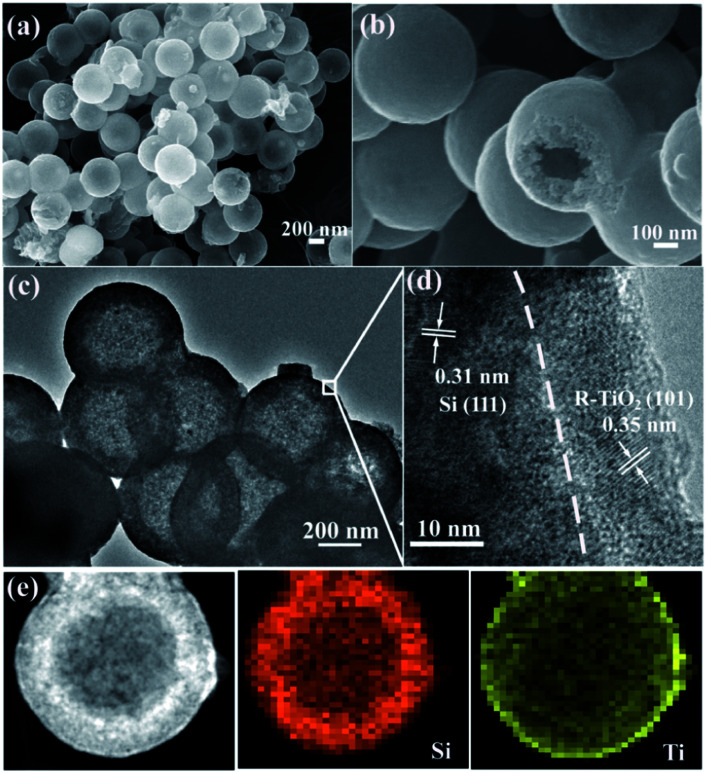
(a and b) SEM images and (c and d) HRTEM images of MHSi@TiO_2−*x*_-800, (e) HRTEM image and energy-dispersive X-ray elemental mapping of Si and Ti of MHSi@TiO_2−*x*_-800.
